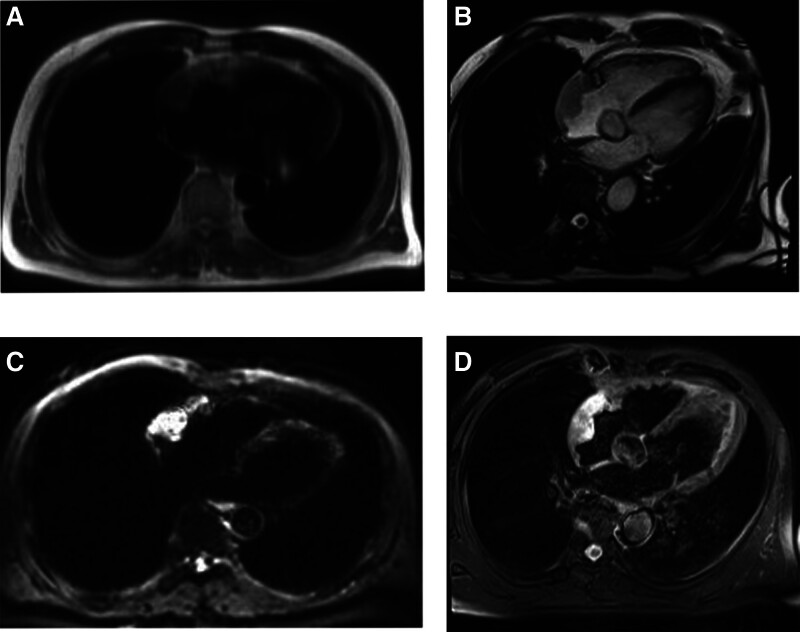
Cardiac MRI examination of the 4-chamber cardiac position. (A) T1WI showed isointensity of the right atrial lesion. (B) The right atrial lesion showed slightly hyperintensity on T2WI. (C) DWI showed high signal intensity in the right atrial lesion. (D) The lesions were significantly enhanced on T1WI + C. The dimensions measured approximately 5.6 × 2.4 cm. MRI = magnetic resonance imaging.
